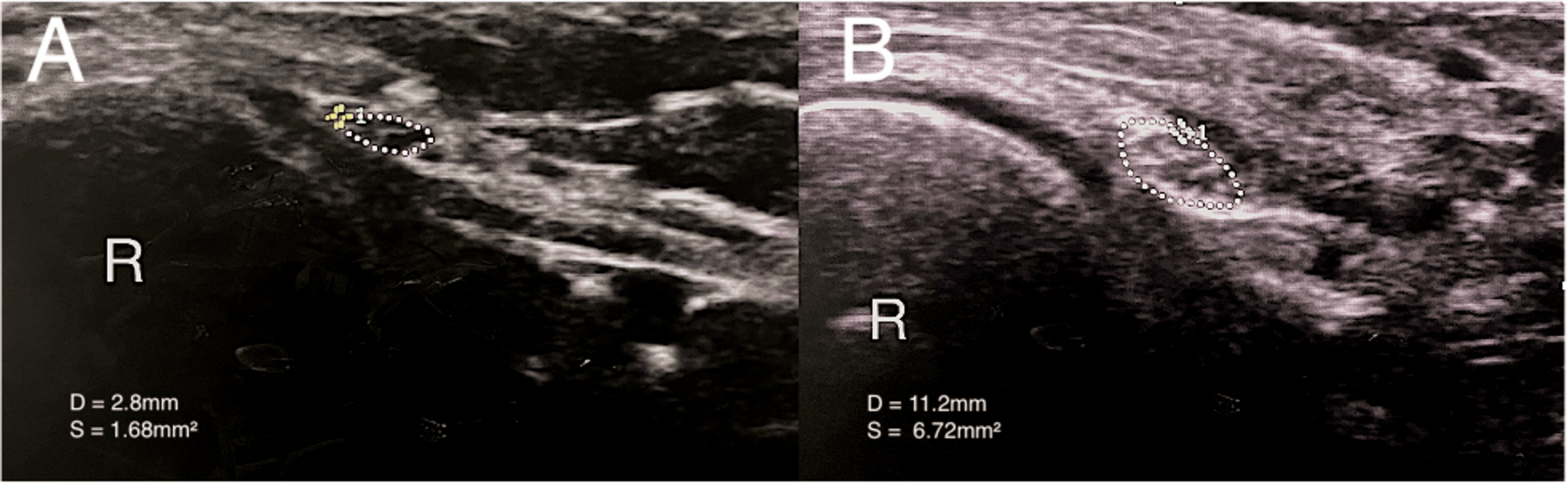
High-resolution ultrasonography images of the superficial radial nerve. A: Normal superficial radial nerve (SRN) on the contralateral side (R = radius), measuring 2.8 mm in diameter with a cross-sectional area (CSA) of 1.68 mm². B: Entrapped SRN on the affected side at the distal forearm scar site, showing marked enlargement (11.2 mm, CSA = 6.72 mm²) and hypoechoic appearance, consistent with entrapment neuropathy.
